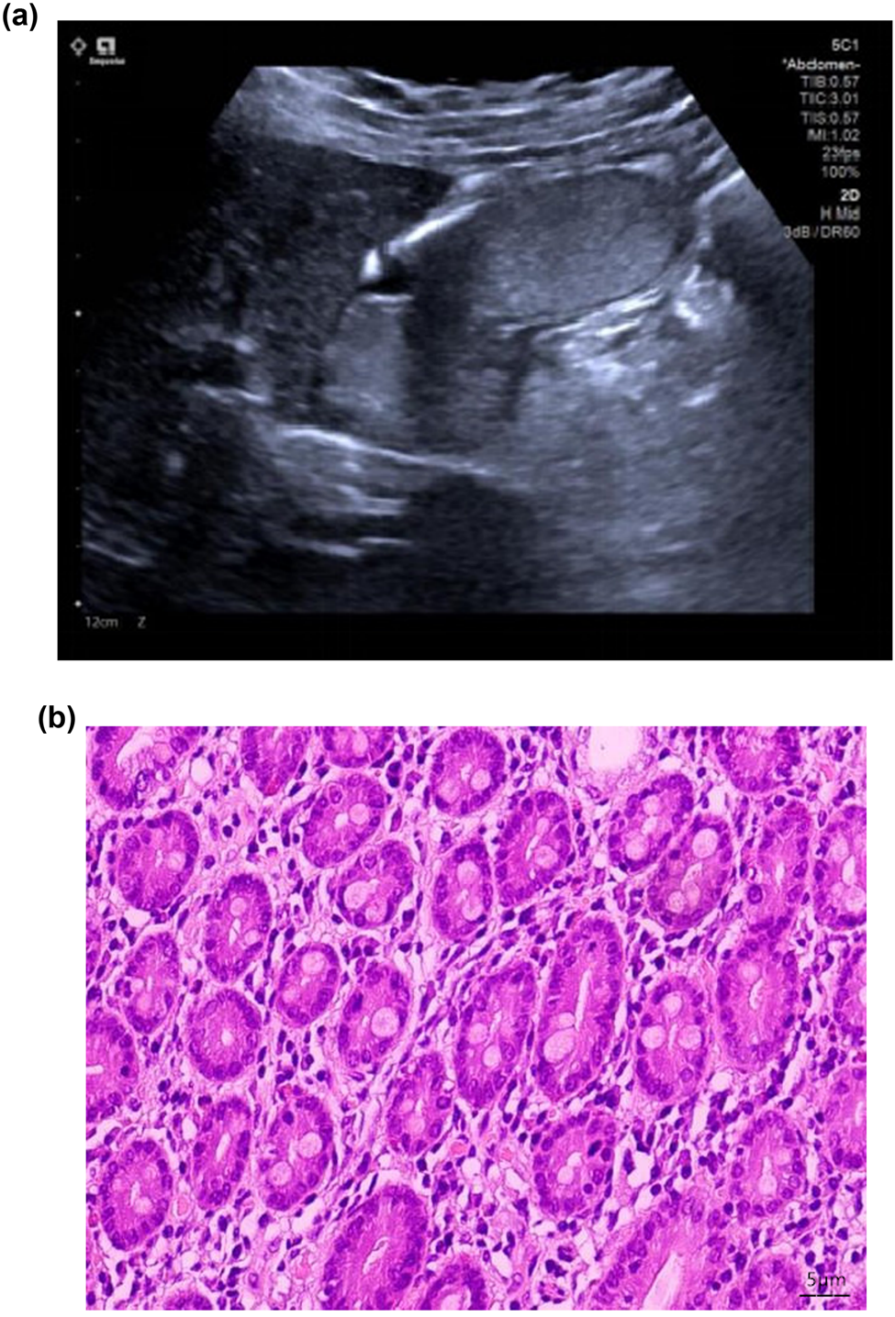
(a) Ultrasonogram of a normal stomach (an ultrasound image of the stomach in a filling state in a healthy person, where the gastric cavity is filled and enlarged, showing liquid echoes with small bubbles floating, and the layered structure of the gastric wall is clearly displayed). (b) Pathological picture of a normal stomach (in a healthy person, the stomach has a normal gastric mucosal layer and gastric glands, and the complete glandular structure can be seen).
